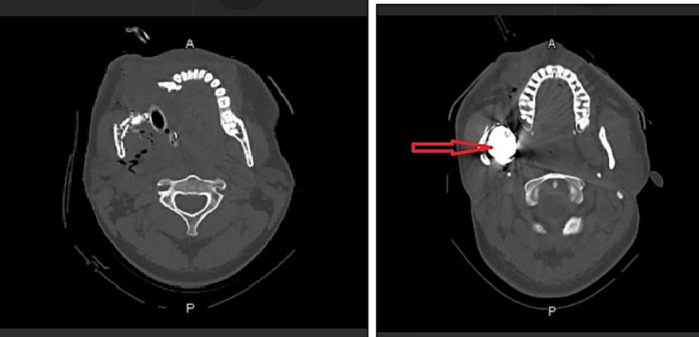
CT scan for case 1 (arrow: foreign body).
